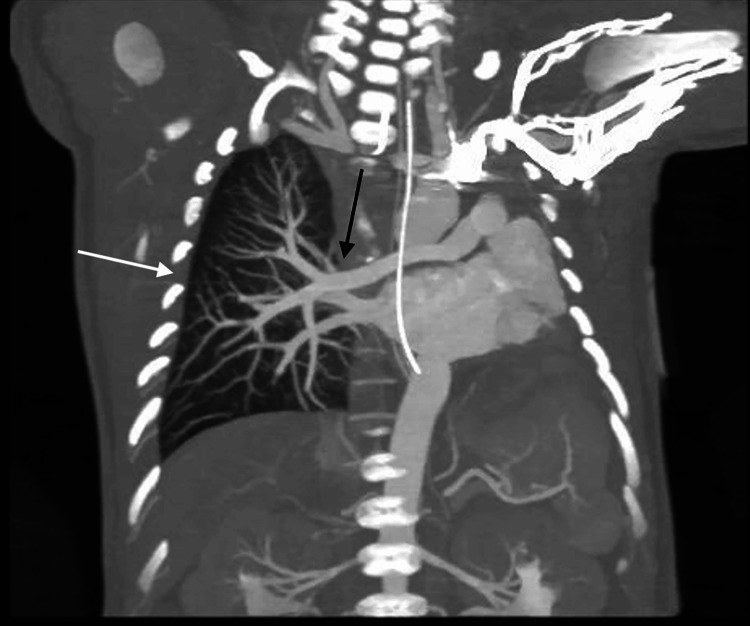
Coronal computed tomography angiography demonstrating left lung agenesis with absent pulmonary arteries and veins The right lung (white arrow) is expanded and receives the entire pulmonary circulation (black arrow).
